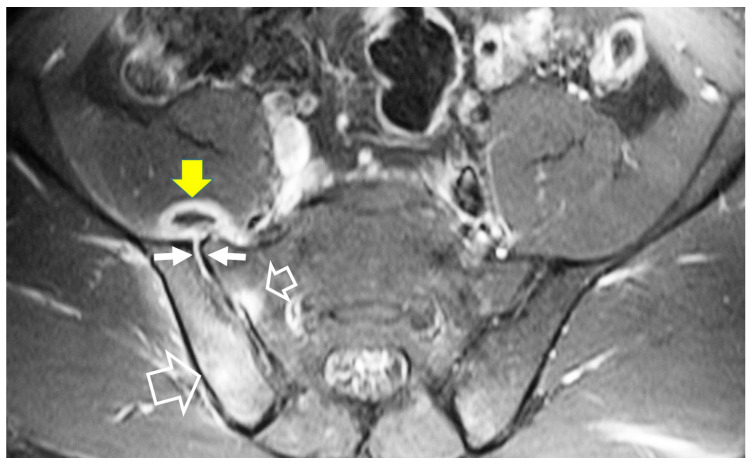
A 23-year-old male with a serologically proven diagnosis of brucellosis 9 months prior to current imaging. The patient received treatment for 3 months and now presents with recurrent symptoms. Axial fat-suppressed T1-w MR image showing enhancing bone marrow edema on both sides of the sacroiliac joints (open arrows), joint effusion (arrows), and anterior capsular thickening and enhancement (yellow arrow) in keeping with septic sacroiliitis.
